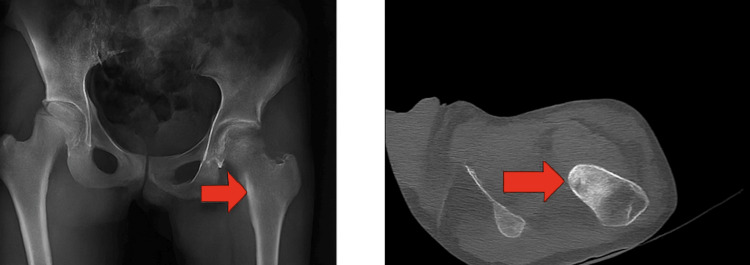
Preoperative AP Pelvic Radiograph and Axial CT Scan Showing Osteoid Osteoma AP pelvic radiograph and axial CT image demonstrate a radiolucent nidus with surrounding sclerosis in the femoral neck, typical of intra-articular osteoid osteoma. AP, anteroposterior; CT, computed tomography.
